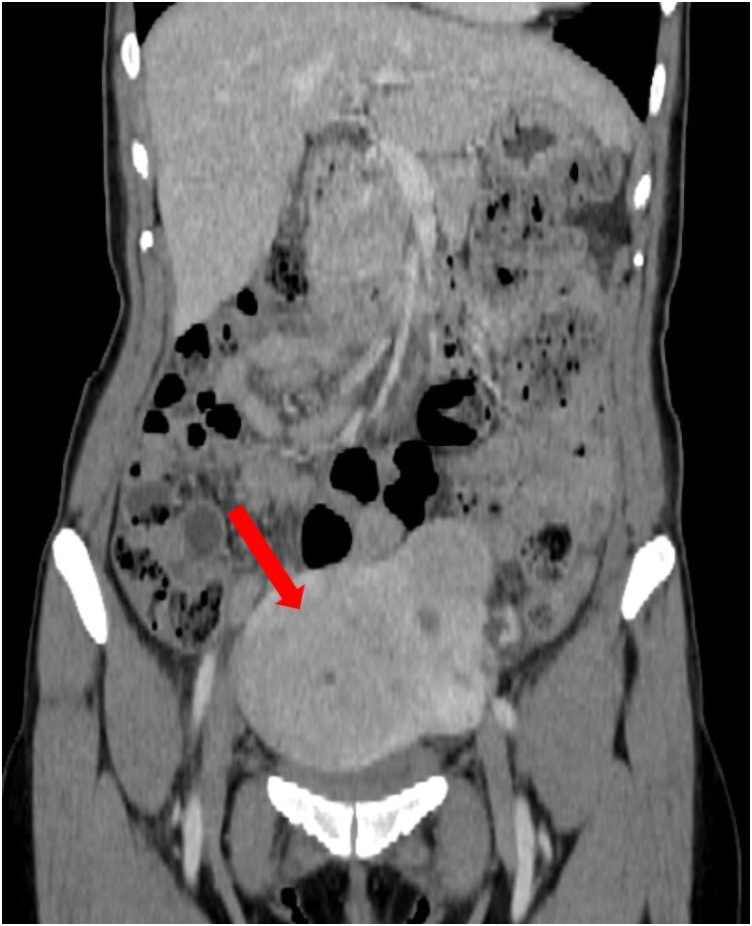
Computed tomography image showing a large pelvic mass measuring approximately 10 cm in size.
